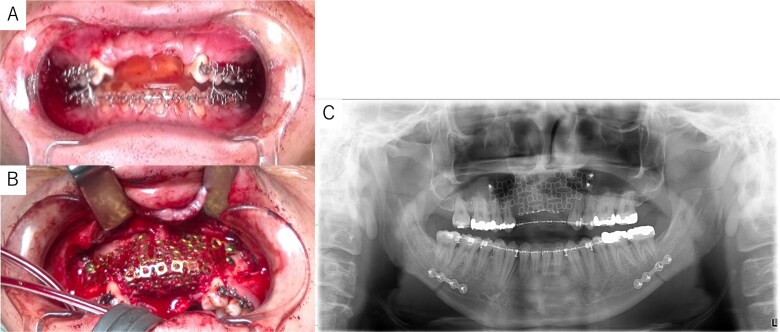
Intraoperative and postoperative images. (A) SSRO surgery was performed with conformity to the orthodontist-prepared final splint. (B) PCBM was grafted into the bone defect and covered with titanium mesh. (C) Postoperative panoramic radiograph showed no abnormalities.
